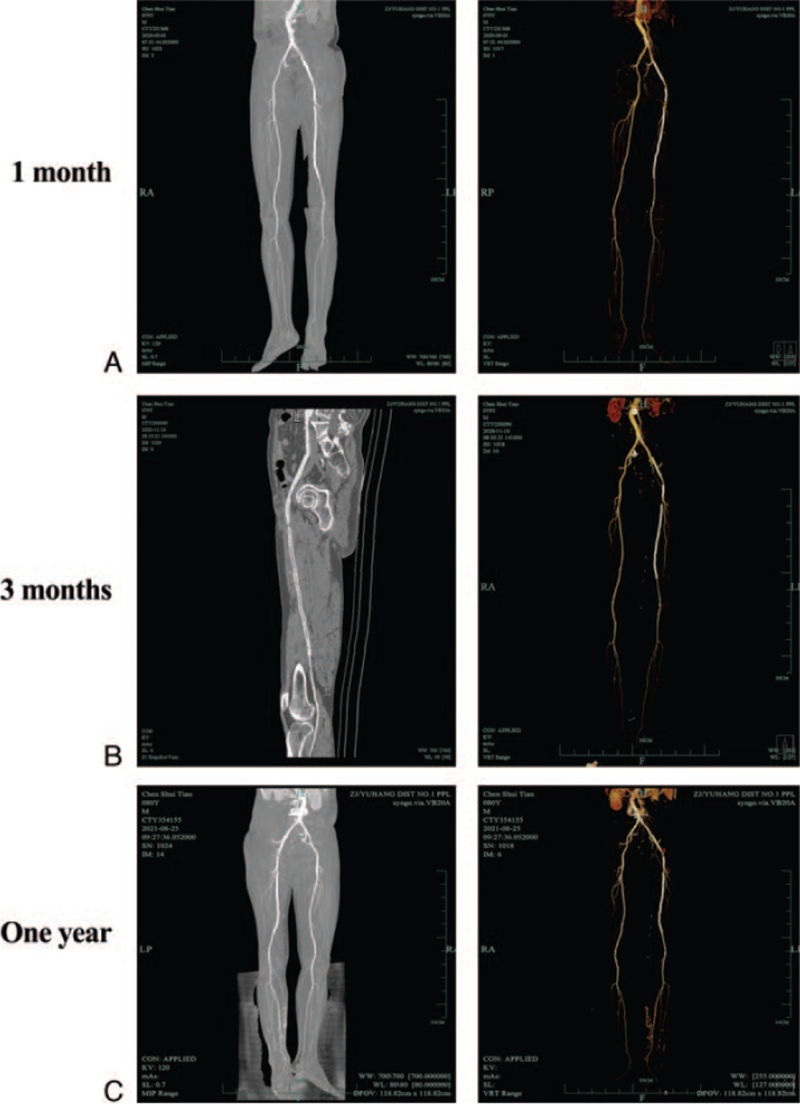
C D&E The post-operative CT images at 1 month, 3 months, and 1 year after dual surgeries, the results of successful cannulation at the lower extremity arteries.
